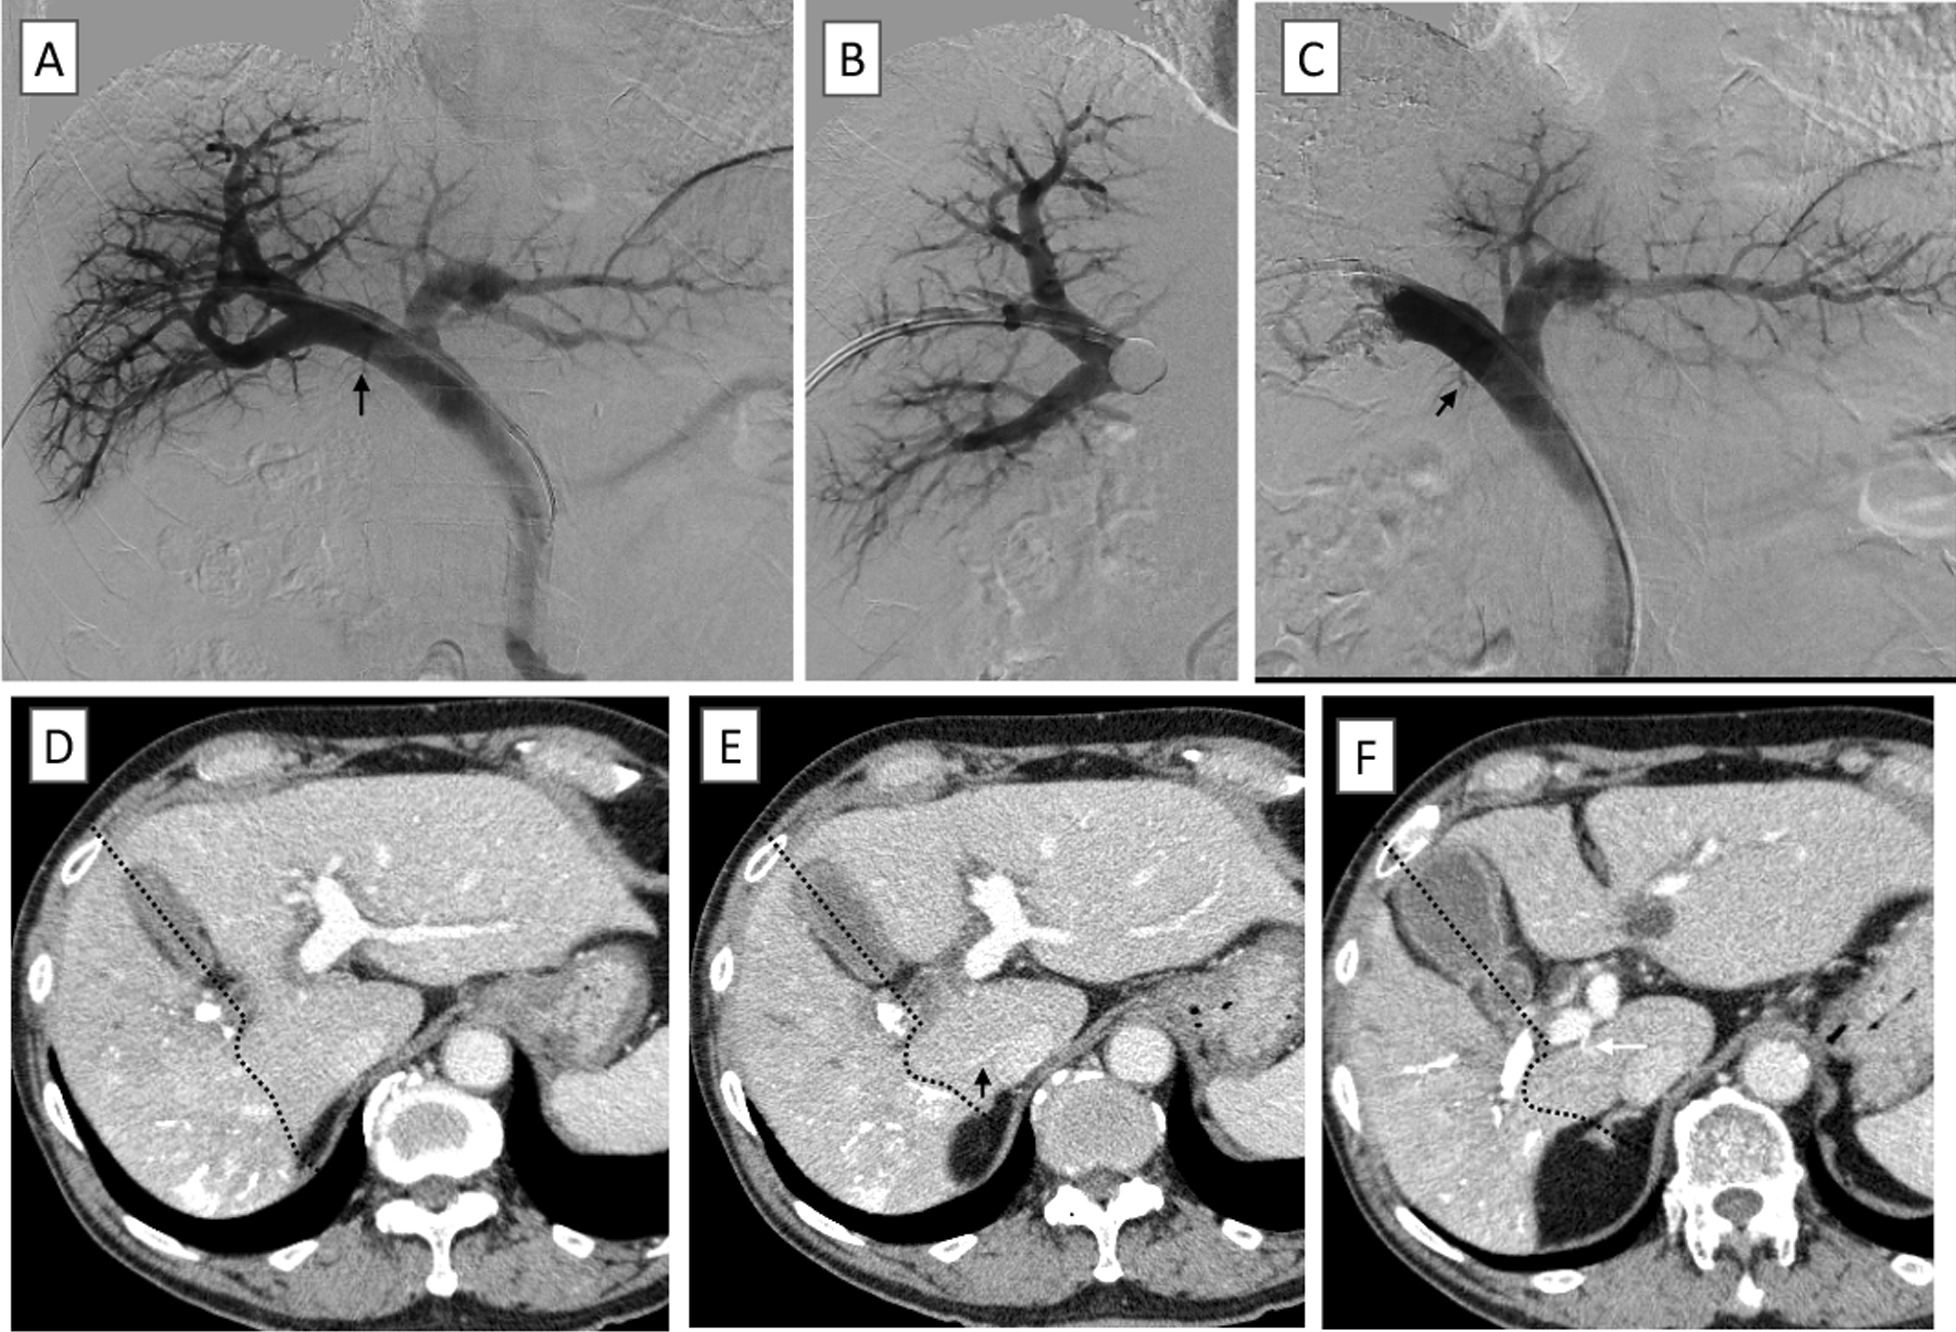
Findings of portgraphies during portal venous embolism (PVE) and enhanced CT findings 18 days after PVE suggestion. A Portography showing the right caudate lobe branch of the portal vein (PV1R) branching from the root of the right portal vein. B Imaging showing portal venous embolism (PVE). C Portography after PVE showing PV1R is preservable. D–F Enhanced CT on day 18 showing the enlargement of right caudate lobe (Dotted line: planned hepatic resection line, Black arrow: inferior right hepatic vein, White arrow: right caudate branch of the portal vein)
